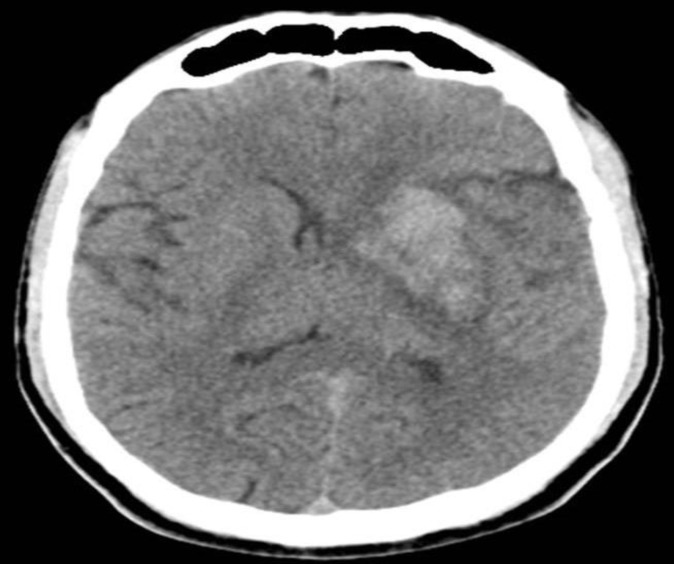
Post thrombolysis CT scan of brain shows hemorrhagic transformation
